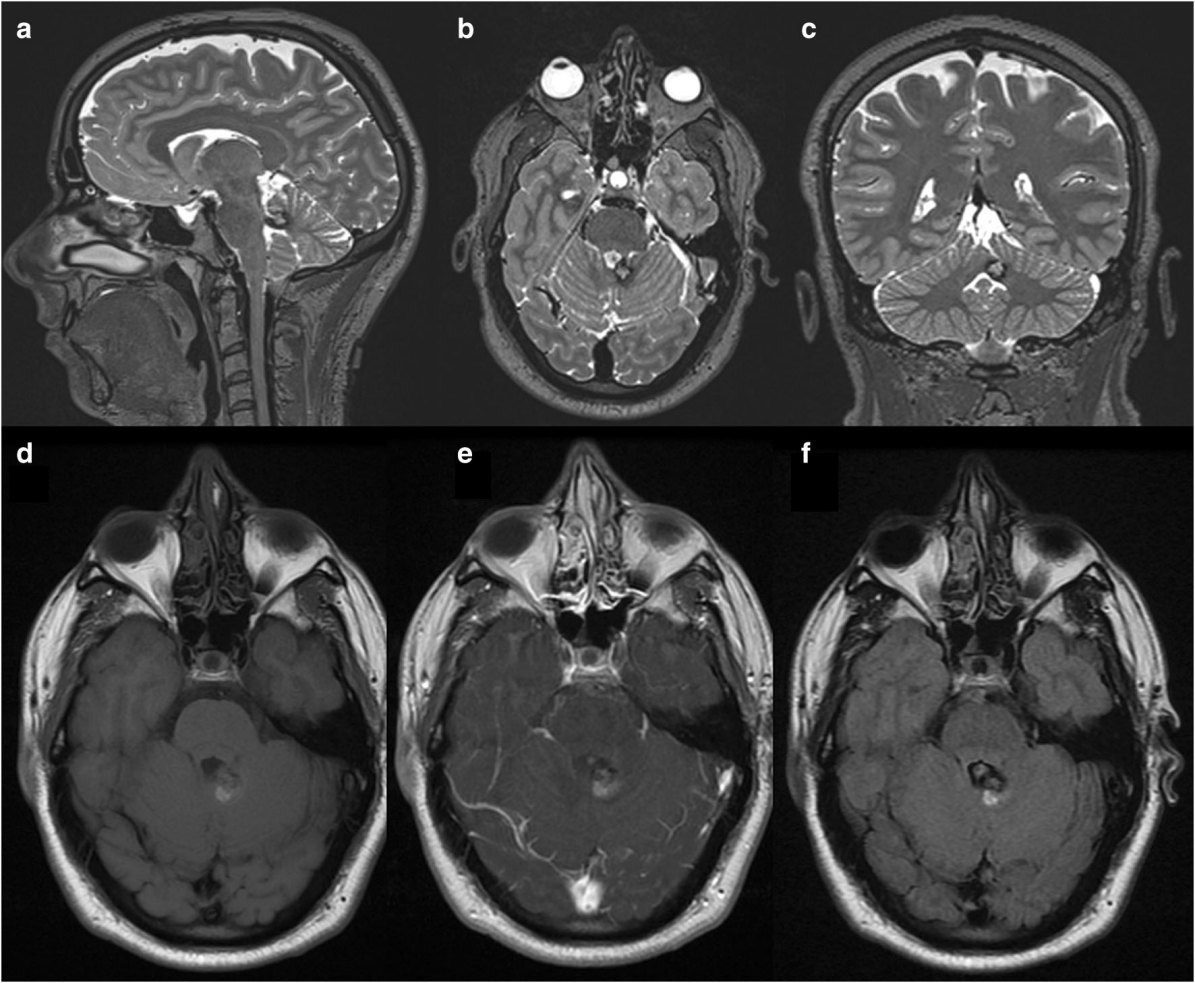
Pre-operative MRI: a–c T2-weighted images revealing the cerebral cavernous malformation on the superior medullary velum with a hypointense ring (Zabramsky type I). T1-weighted images—non-contrast (d) and with gadolinium (e)—and FLAIR (f) supporting acute blood around the cerebral cavernous malformation lesion
